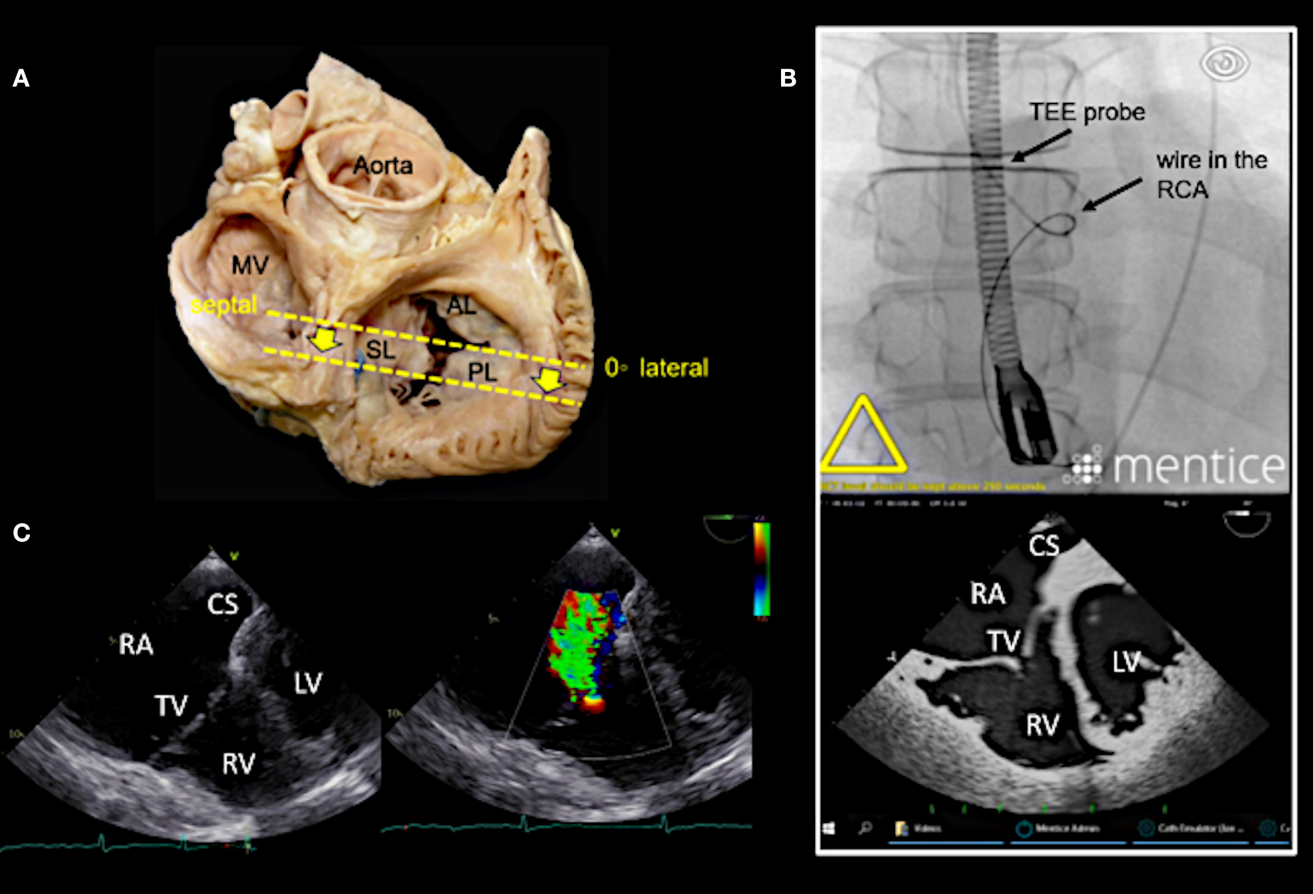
Deep esophageal view at 0–10°. (A) The specific imaging plane for the deep esophageal view is shown (see also Figure 3A). Starting from the mid-esophageal 4-chamber view at 0–10°, the probe is advanced deeper until the CS becomes visible. This maneuver allows for an easy evaluation of the septal leaflet. The evaluation of the posterior region of the septal and the posterior leaflets is of particular importance since the main axis of tricuspid annular dilation is in anterior-posterior direction and leaflet retraction is often pronounced in the posterior part. (B) The upper picture shows the necessary positioning of the TEE probe in fluoroscopy (35° RAO, 6° CRANIAL) to create the deep esophageal view at 0° (see image below). (C) The corresponding 2D TEE view without (left) and with (right) color Doppler is shown. CS, coronary sinus; other abbreviations see Figure 3.
